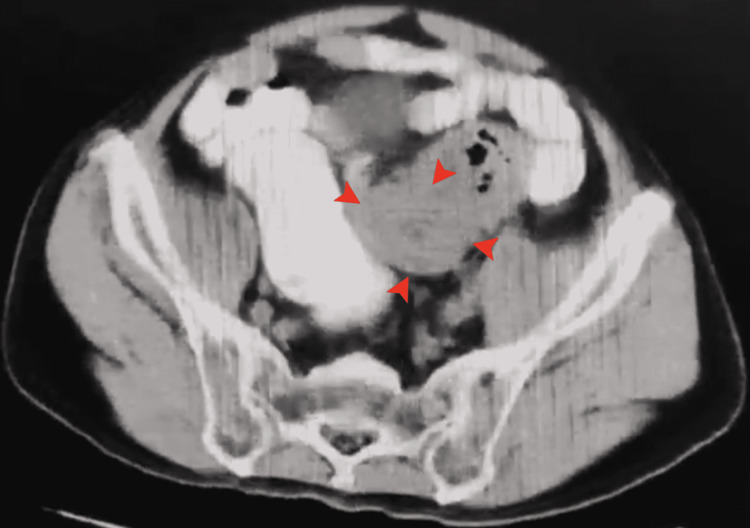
Transverse plain CT scan: arrows point to a concentric obstructive mass measuring 7-8 cm in the sigmoid colon
